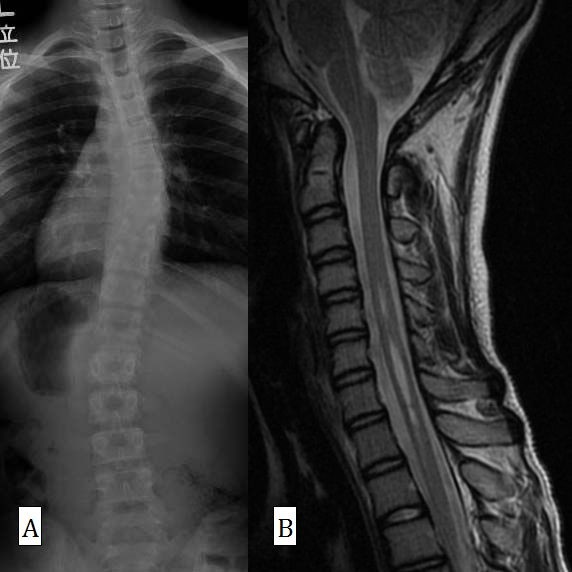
Posteroanterior radiograph (A) and sagittal MRI (B) in Case 9. The patient was neurologically normal. The syrinx was spindle type and the space of the cisterna magna was preserved. This patient was not suitable for FMD and was treated using an underarm brace.
